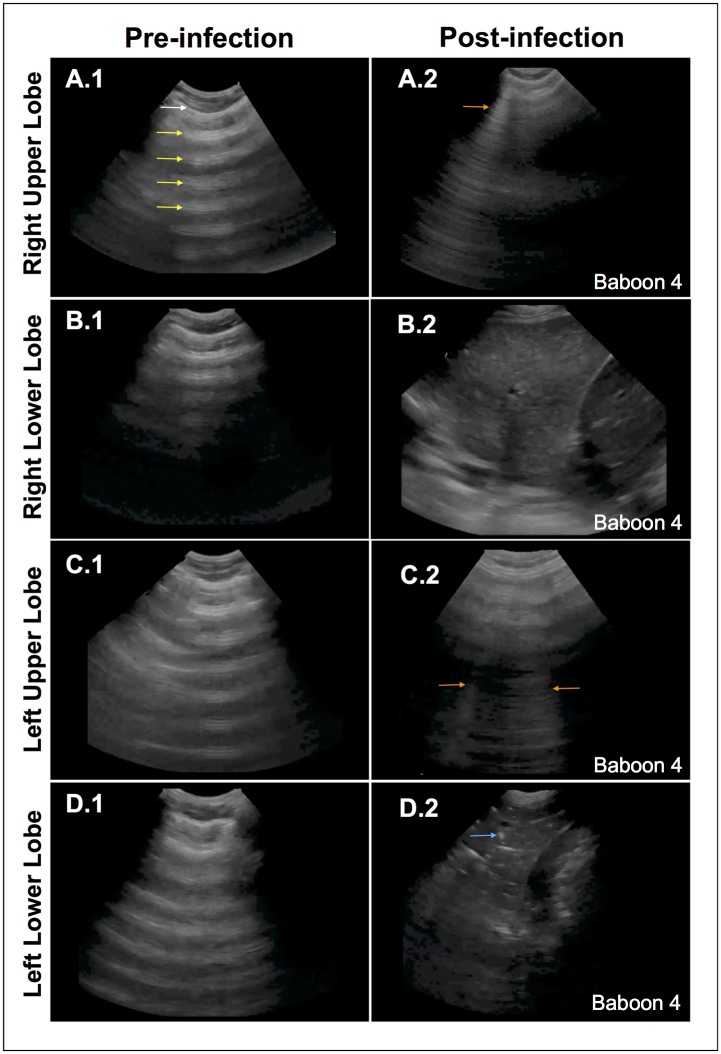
Ultrasonography diagnosis of pneumonia. Representative images of baseline ultrasonography (A.1-D.1) demonstrate normal lung findings: normal pleura (*white arrow*) with reverberations of the pleura, or A-lines, seen in the air-filled lung (*yellow arrows*). The end-of-experiment images demonstrate different pathologic findings. The right upper lobe (A2) shows a discrete, vertical hyperechoic line, or B-line (*orange arrow*), a type of reverberation artifact due to interlobular septal edema. The right lower lobe (B2) shows a densely consolidated lobe that has similar echogenicity as the liver (“hepatization”) due to replacement of lung air with fluid. The left upper lobe (C2) shows a few B-lines (*orange arrows*) due to interstitial edema. The left lower lobe (D2) shows a densely consolidated lobe with distinct white speckled areas that are air-bronchograms due to the air-water interface in the terminal bronchioles (*blue arrow*). A small pleural effusion (*) is also seen.
